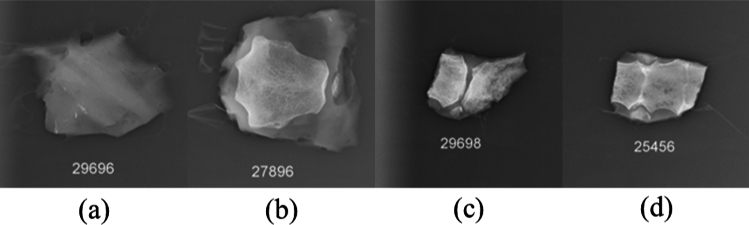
X-ray images for composition. a shows the sample doesn’t contain bone, primary cartilage or soft tissue. b shows the sample partially contains bone. c and d show samples are primarily bone
